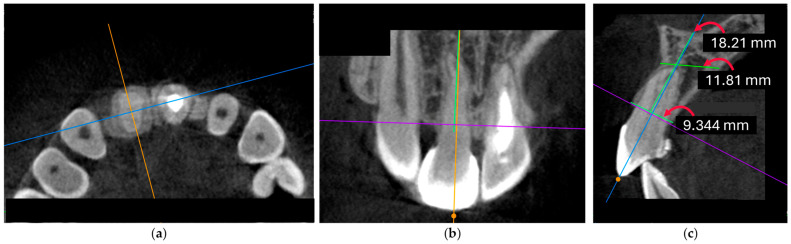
Axial (a), coronal (b), and sagittal (c) CT scans taken 7 years after trauma, at the 5-year post-transplantation follow-up, May 2026. The height of the alveolus increased by 5 mm. Subfigure (c) highlights in green the dimensions of the alveolar bone.
